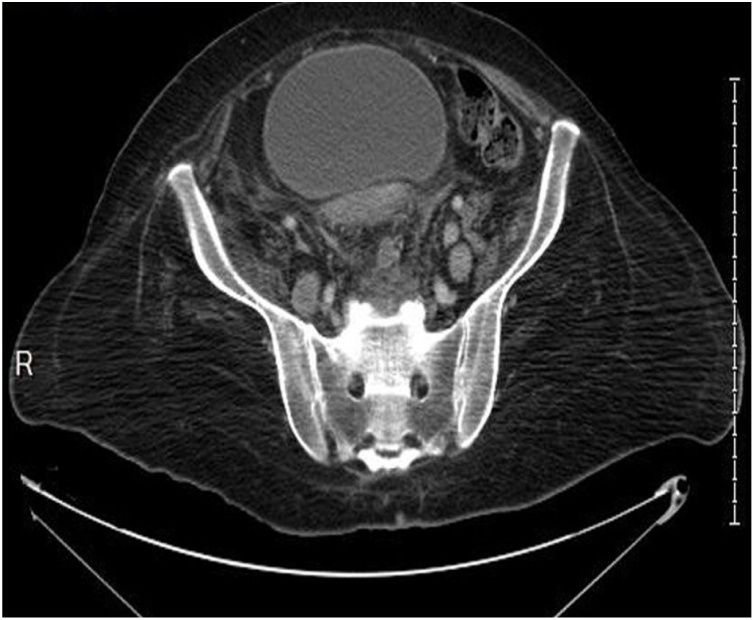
Axial CT scan of the pelvis shows multiple enlarged lymph nodes seen along the iliac vessels.
